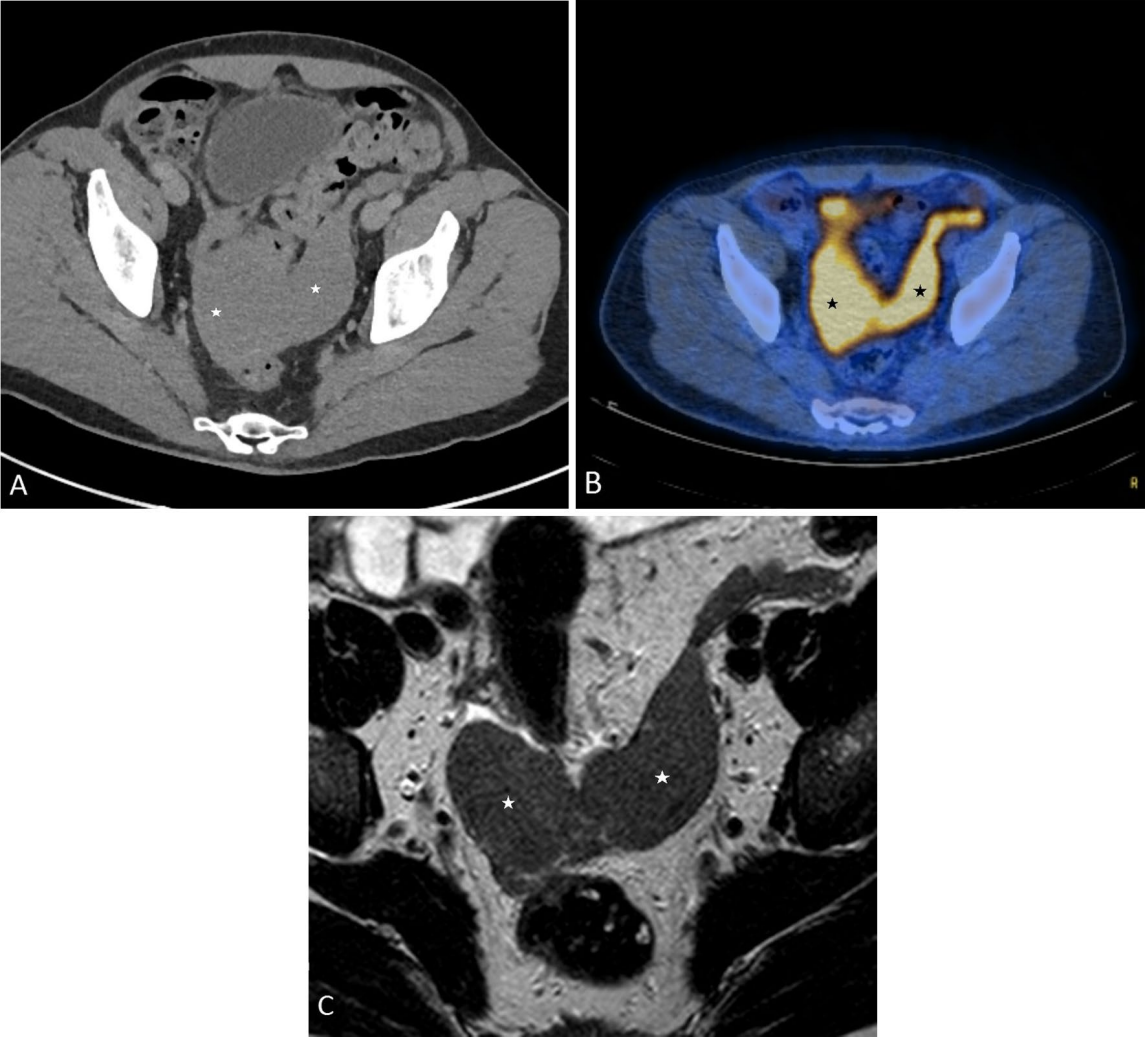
a–c 48-year-old male with ‘dry’ appearance of epithelioid type of malignant peritoneal mesothelioma. a, b Axial PET-CT images through the pelvis showing omental caking (*), nodular mesenteric fold thickening (arrows). b Axial image through the pelvis showing soft tissue density, FDG-avid, plaque-like, nodular soft tissue thickening of the pelvic peritoneum (*). c MRI T2 axial image showing T2 intermediate signal intensity, nodular soft tissue (*) along the pelvic peritoneum
